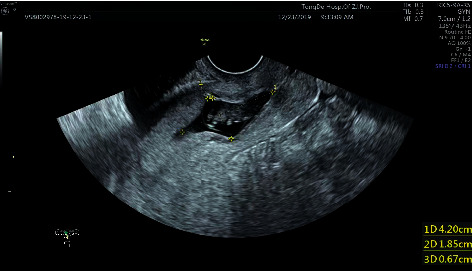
B ultrasound acoustic image of a 38-year-old CSP patient who presented with amenorrhea for 59 days and vaginal bleeding along with low back pain for 10 days. The gestational sac was located on the scar on the anterior wall of uterine isthmus with pulse of the primitive heart tube. The size of the gestational sac is 4.2 × 1.9 × 2.8 cm.
